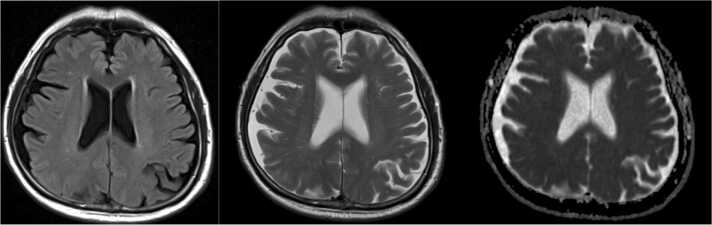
The initial MRI on presentation had no abnormal findings.
